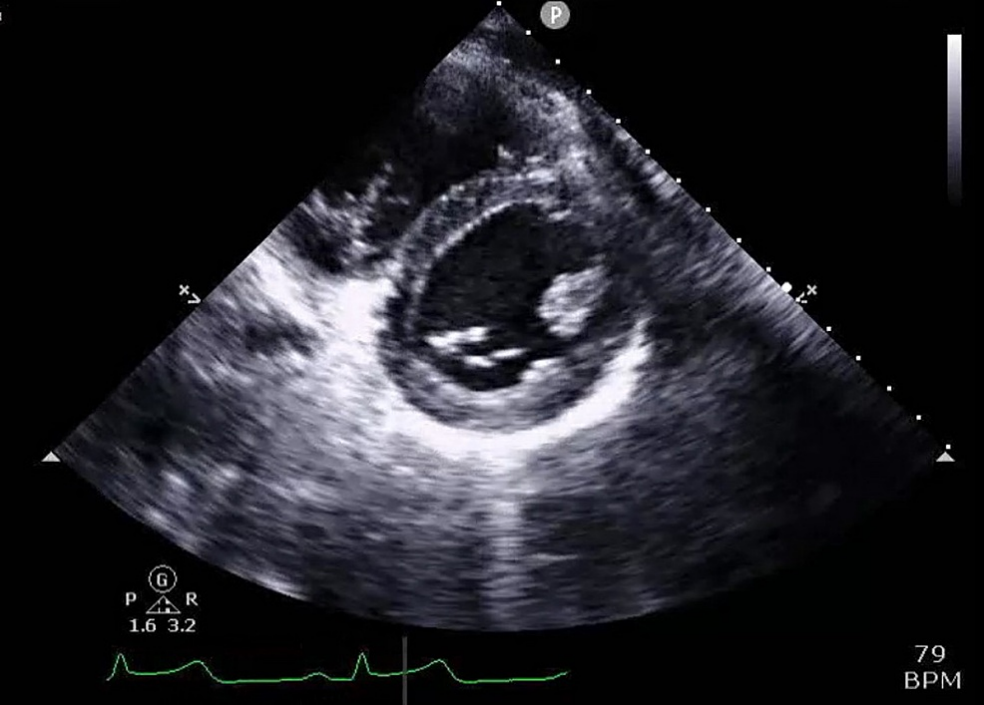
Parasternal short axis trans thoracic echocardiogram image
